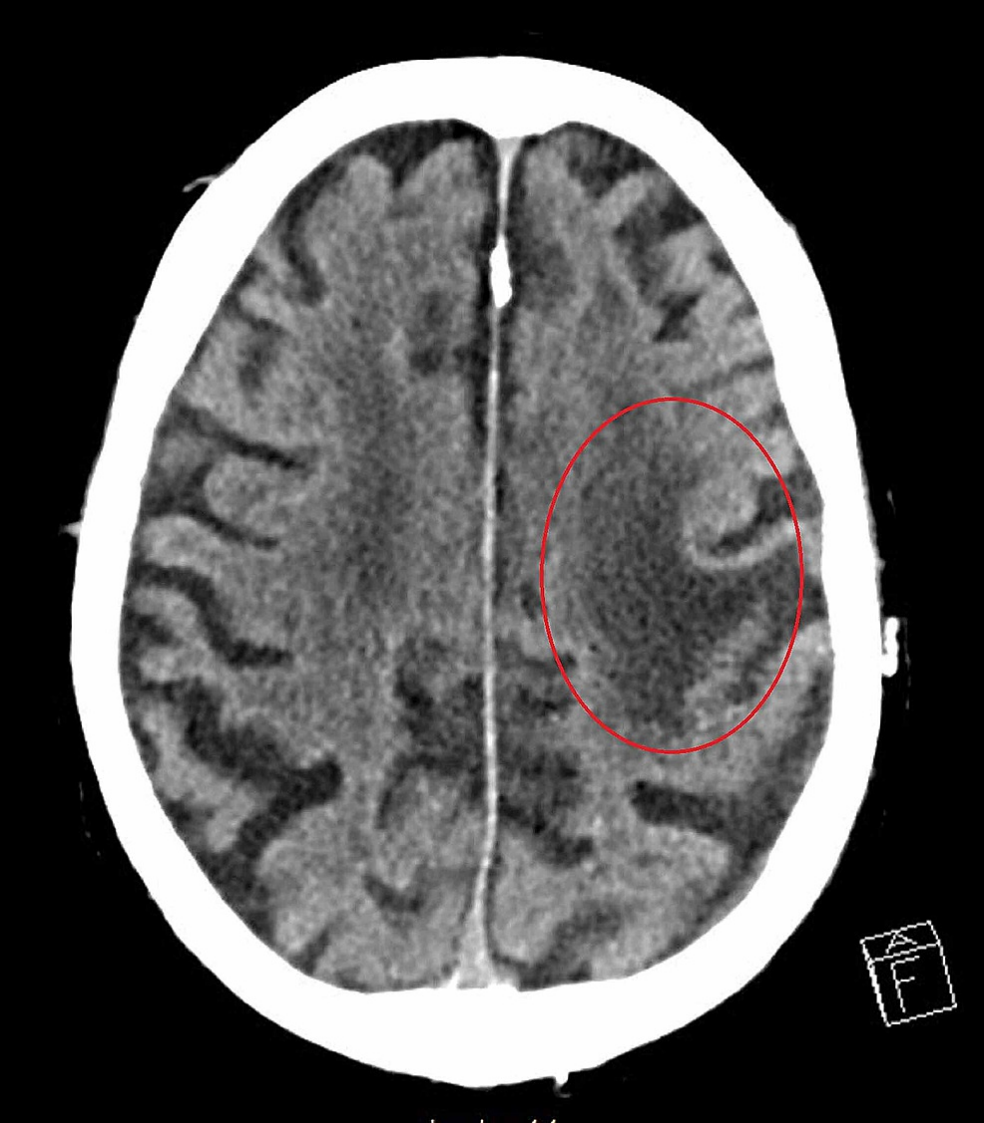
NCCT scan of head showing hypodensity in left frontal lobe representing chronic encephalomalacia. NCCT = non-contrast computed tomography
